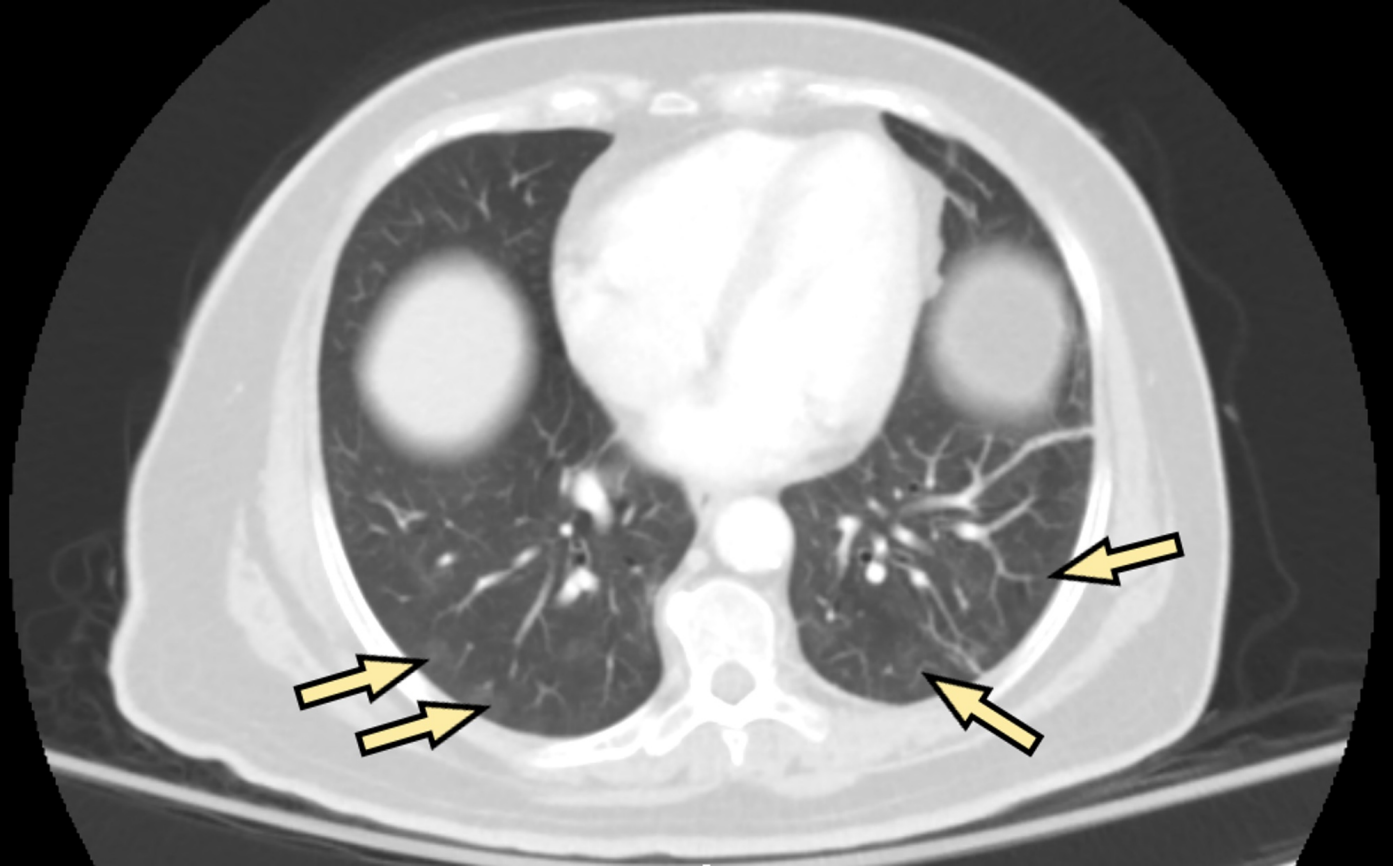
Bilateral peripheral ground-glass opacities in the lung bases (arrows) of a 61-year-old woman, unexpectedly noted on axial abdominopelvic CT performed to assess the extent of a perianal abscess.
